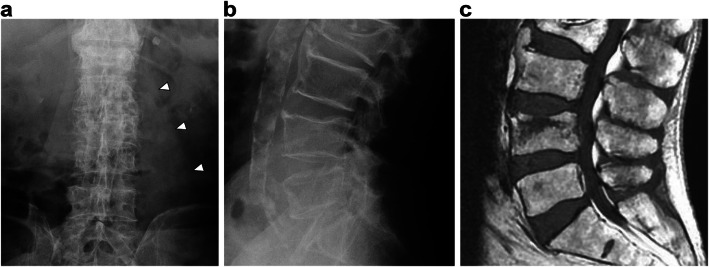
X-ray images and magnetic resonance imaging (MRI) of lumbar spine on the day of admission. a,b X-ray shows thickened left psoas major muscle (arrowhead) and no evidence of an unstable spinal fracture. c T1-weighted MRI shows L4 VCF estimated as a stable-type spinal injury considering that only the anterior column, and not the middle or posterior column, was injured
